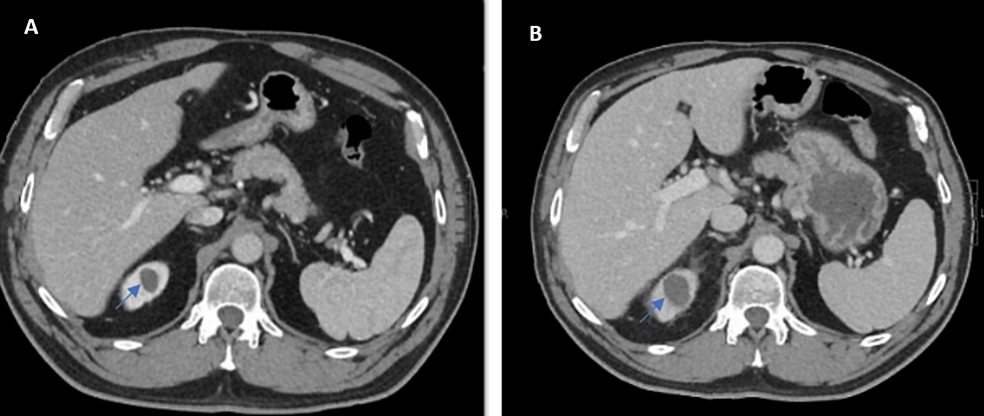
(A) CT scan showing an 18 mm right renal cyst (three years before presentation); (B) CT scan showing a 26 mm right renal cyst in addition to increased density and perinephric fat stranding (at the time of presentation).
